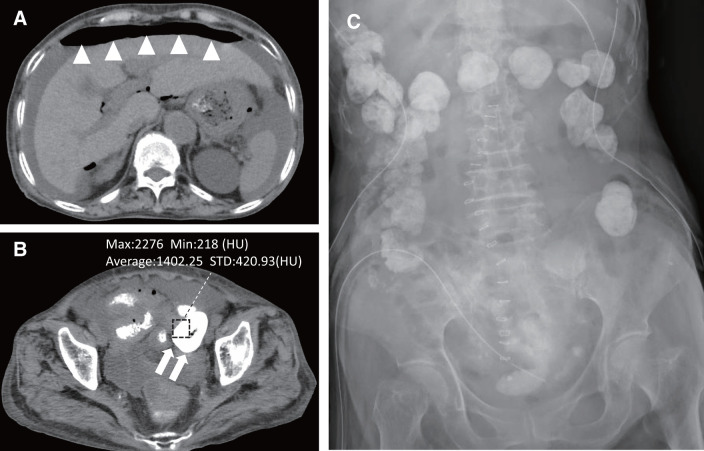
CT images at the time of admission and postoperative X-ray. Presence of massive free air patches (white triangles) in the upper abdominal area (A) and high-intensity feces in the extraintestinal lumen (white arrows) in the pelvic cavity (B) were revealed on abdominal CT imaging with HU. The postoperative X-ray image revealed a massive accumulation of SCZ in the feces (C). CT, computed tomography; HU, Hounsfield units; SCZ, sodium zirconium cyclosilicate
